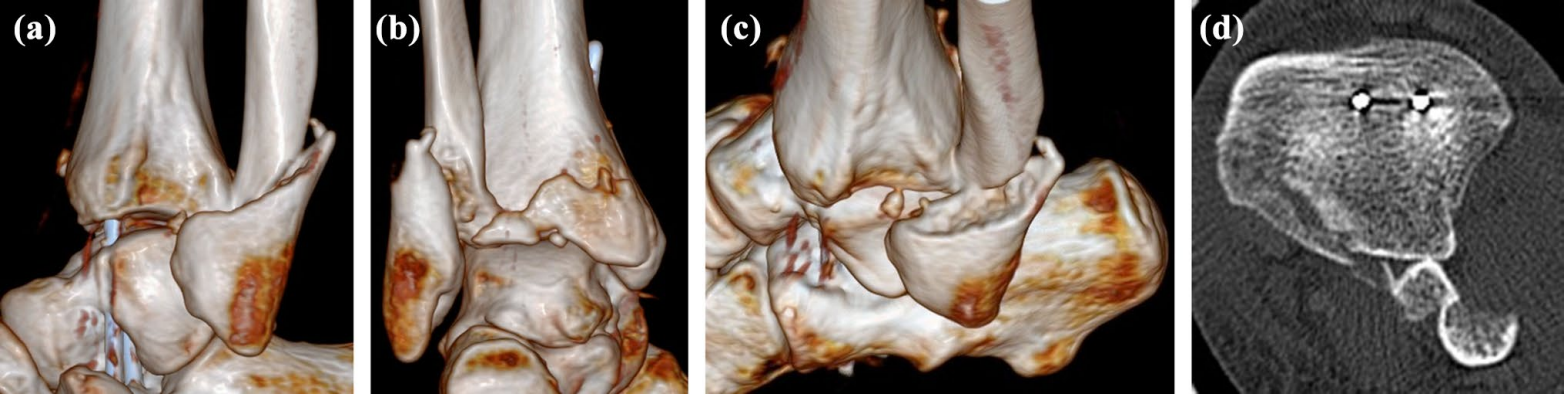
CT imaging demonstrated posterior displacement of the proximal fibular fragment fracture behind the distal tibia and a minimally displaced fracture of the posterior malleolus (Bartoníček-Rammelt type 3, two-part fracture with extension into the medial malleolus). a lateral 3D CT view; b posterior 3D CT view; c lateral 3D CT view; d axial CT scan
